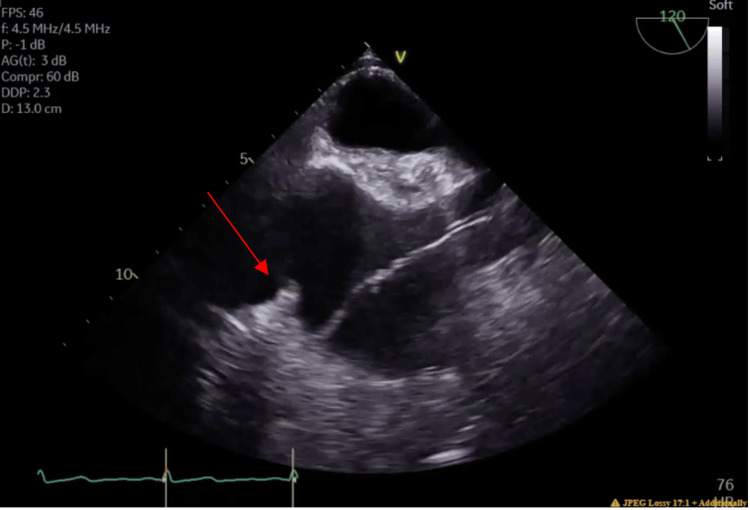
Transesophageal echocardiogram demonstrating 0.8 x 0.2 cm mobile echodensity (red arrow) attached to the eustachian valve.
